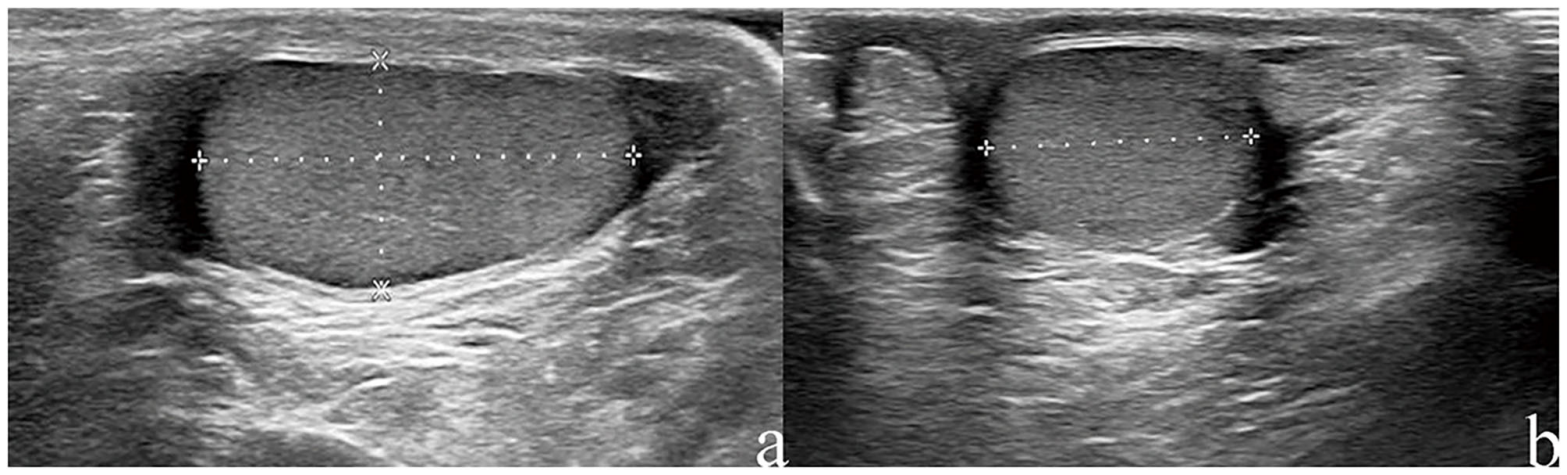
Measurement of testicle length, width, and height by Ultrasound on a 7-years old boy. The dotted lines show measurement of length and height in the longitudinal plane (a) and width in the transverse plain (b).
